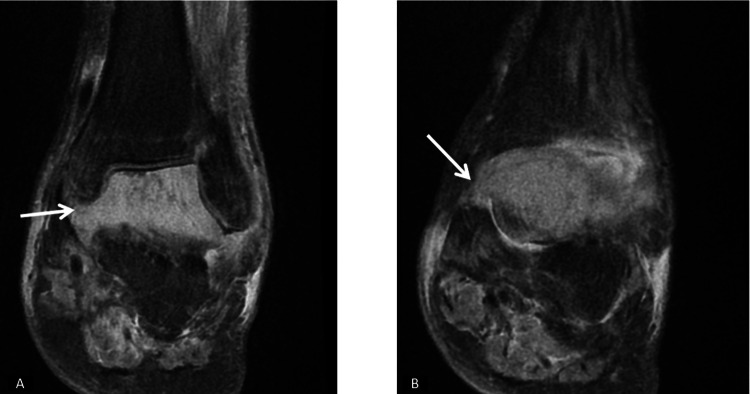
Coronal MRI of the left ankle, obtained with T2-weighted sequences following contrast administration, demonstrates abnormal signal intensity in the ankle with adjacent soft tissue involvement, indicative of potential malignancy. (A) shows involvement around the talus, while (B) highlights involvement in the soft tissue surrounding the talus. The white arrows indicate the area of the lesion. MRI: magnetic resonance imaging
